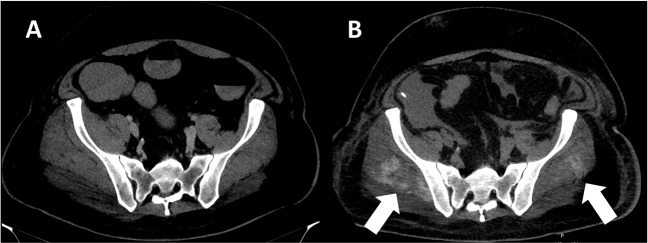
Computed tomography on admission (panel A) and 2 weeks later (panel B). White arrows indicating radiopaque depots of precipitated calcium within gluteal muscles
